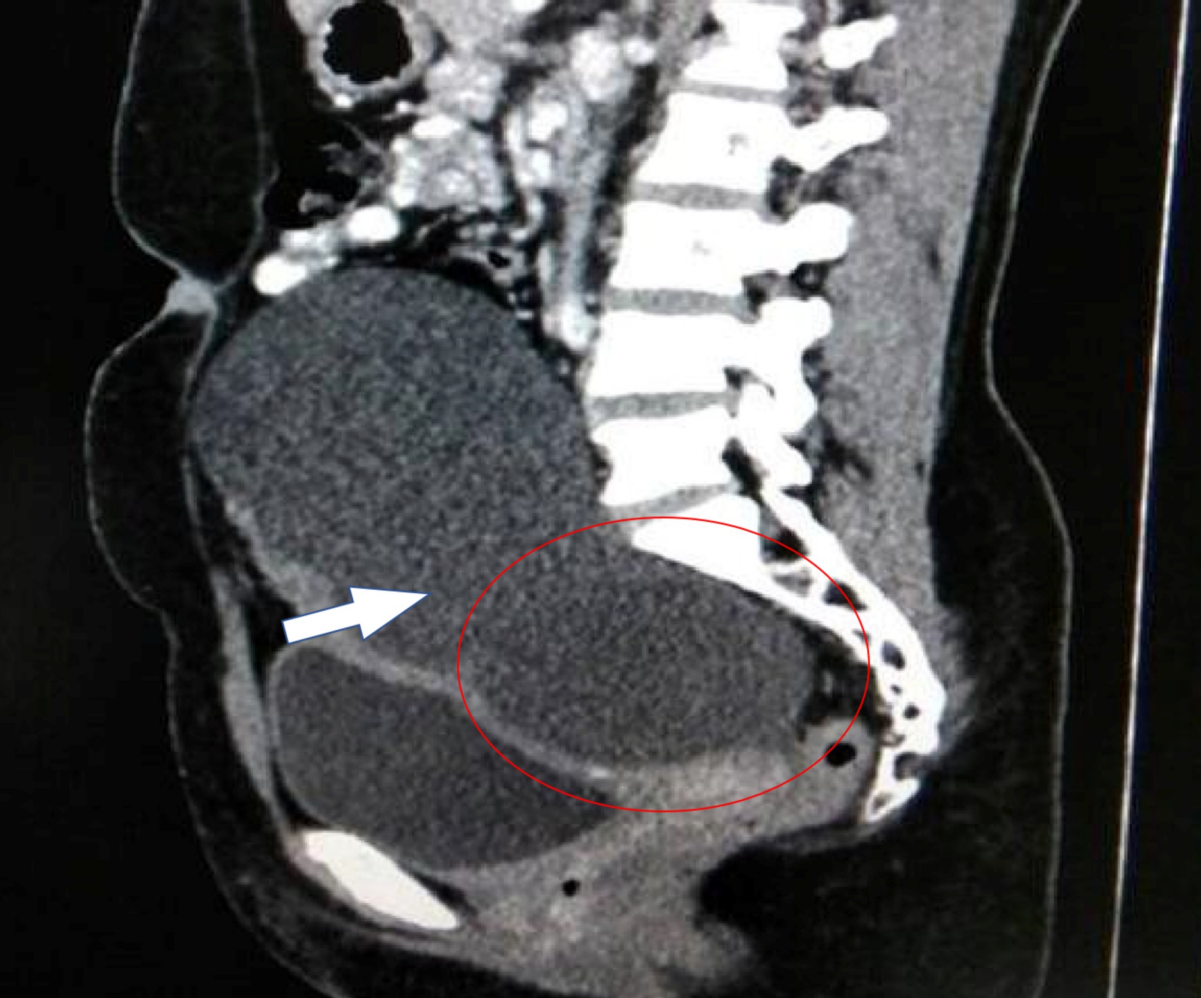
Contrast-enhanced CT scan of pelvis showing cyst arising from Douglas’ pouch.
